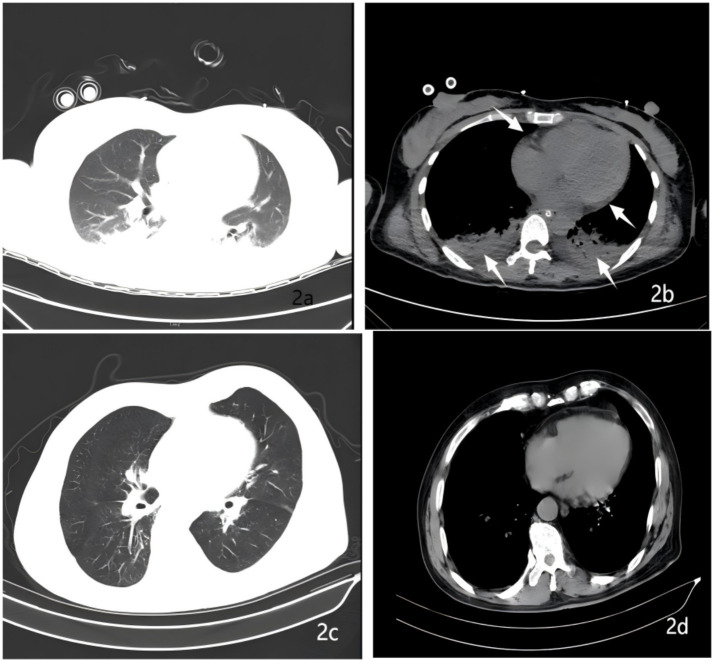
Pulmonary CT findings of the patient. (a) Bilateral pulmonary exudation on postpartum day 3. (b) Bilateral pleural effusion and pericardial effusion on postpartum day 3. (c) Reduced pulmonary exudation on postpartum day 7. (d) Improved pleural effusion and pericardial effusion on postpartum day 7.
